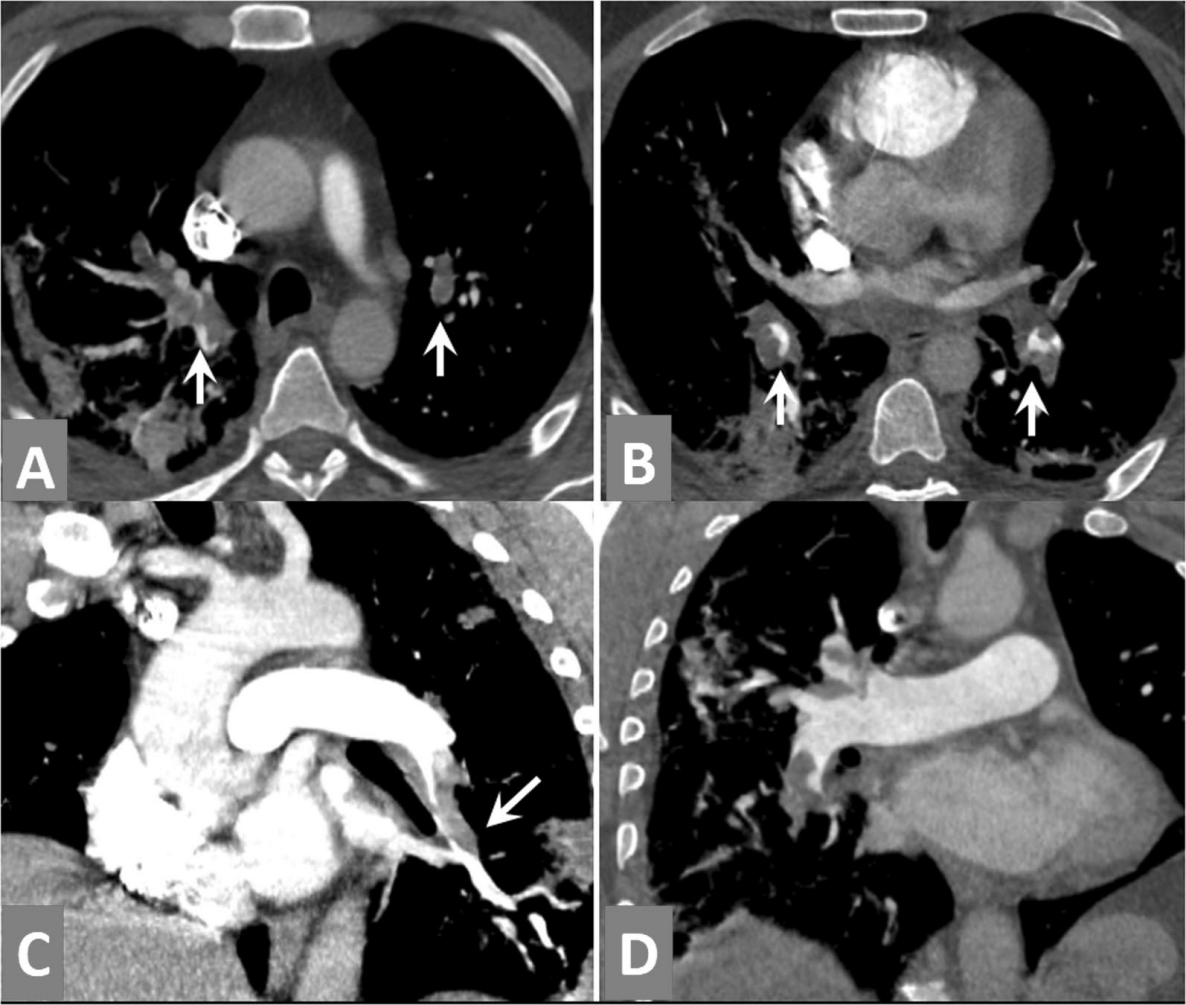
CT-pulmonary angiography (CTPA) examination for a 74-years-old COVID-19 male patient complained of severe chest pain and sudden deterioration of oxygen saturation at the third week of infection. [A, B] Axial CTPA images showing bilateral upper and lower lobar central contrast filling defects together with sub-pleural consolidative atelectatic patches. [C] Coronal CTPA image with MIP reconstruction showed left lower lobar large contrast filling defect extending to the peripheral arterioles with nearby sub-pleural wedge-shaped patch. [D] Coronal CTPA image showing similar right upper lobar changes … Massive pulmonary embolism and pulmonary infarctions
